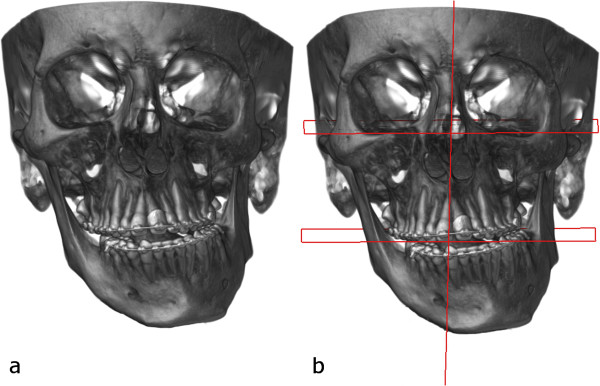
Preoperative CT scan (a) with planes defined (b).
